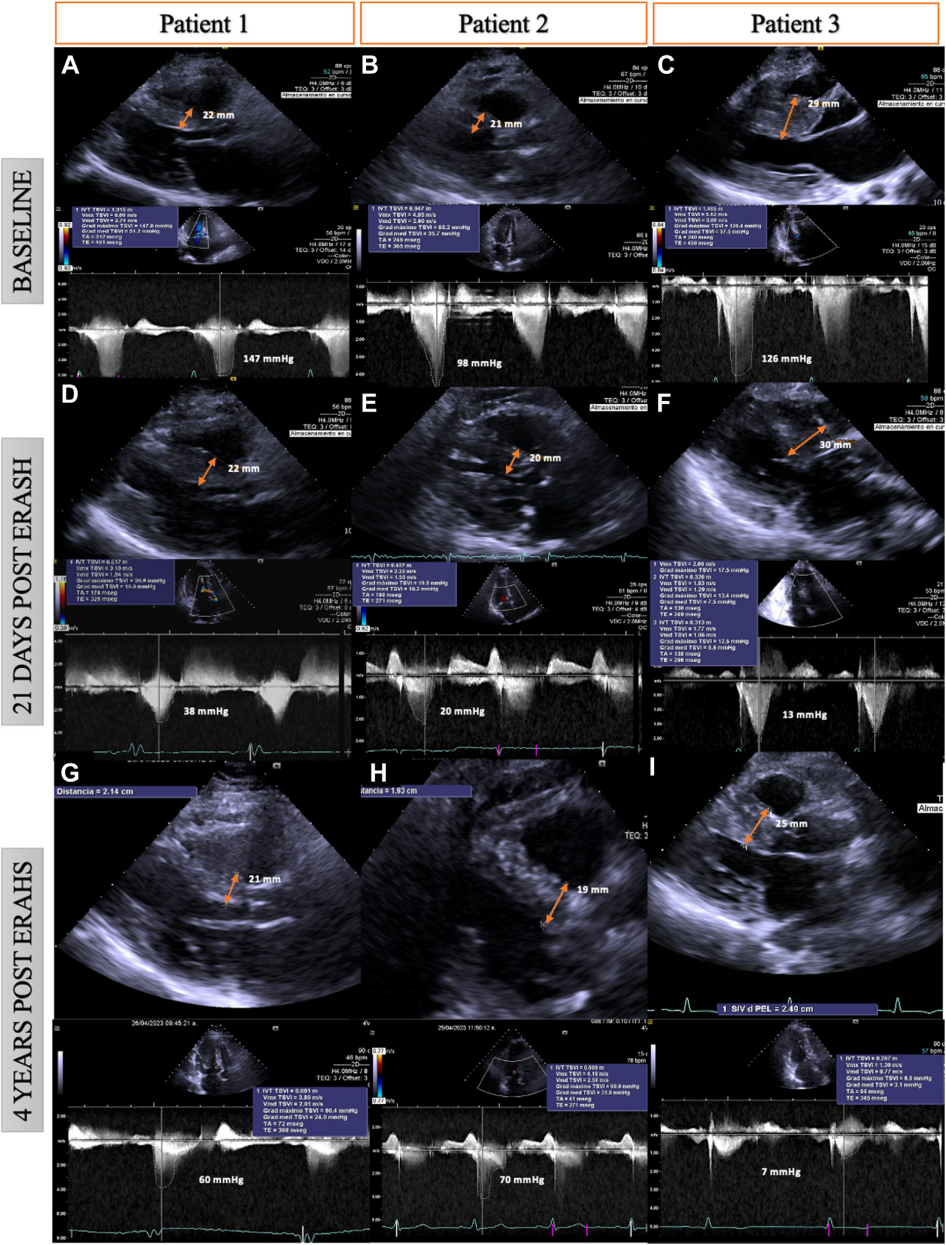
Echocardiographic measurements of septal thickness and LVOT gradients performed under resting conditions before ERASH (A: Case 1; B: Case 2; C: Case 3), at 21 days’ follow-up (D: Case 1; E: Case 2; F: Case 3) and 4 years post-ERASH (G: Case 1; H: Case 2; I: Case 3). ERASH = endocardial radiofrequency ablation of septal hypertrophy; LVOT = left ventricular outflow tract.
